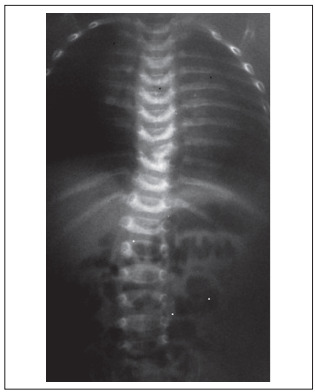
Spinal radiographs showing T9 and T10 hemivertebrae.
